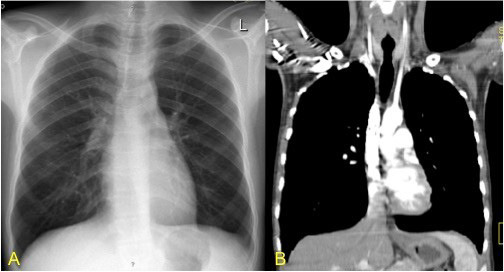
A. The postero-anterior plain chest radiograph and the coronal
